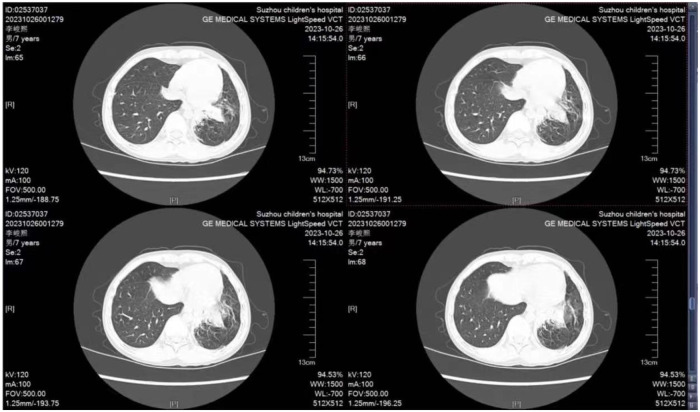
Chest high-resolution CT (2023-10-26) with visible slice and cable-like high-density shadows in both lungs, with the left lower lobe.
